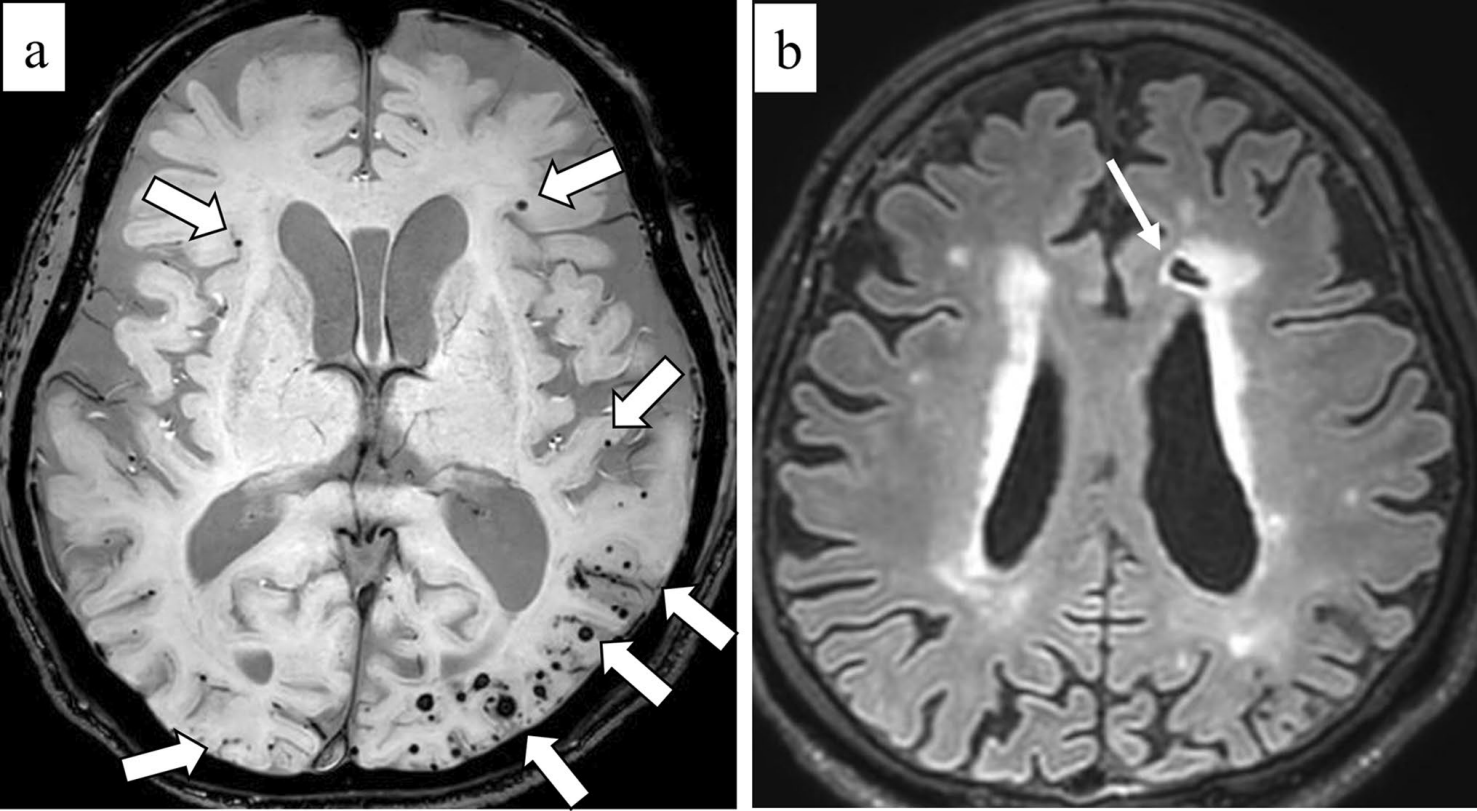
A 75-year-old male with probable CAA. Mini-Mental State Examination (MMSE) score is 20 out of 30. Susceptibility-weighted image (SWI) (a) shows multiple strictly lobar cerebral microbleeds (CMB) (arrows) in bilateral hemispheres with a posterior dominant distribution. Three-dimensional (3D) fluid-attenuated inversion recovery (FLAIR) (b) shows lacune (small arrow) in the left frontal lobe
